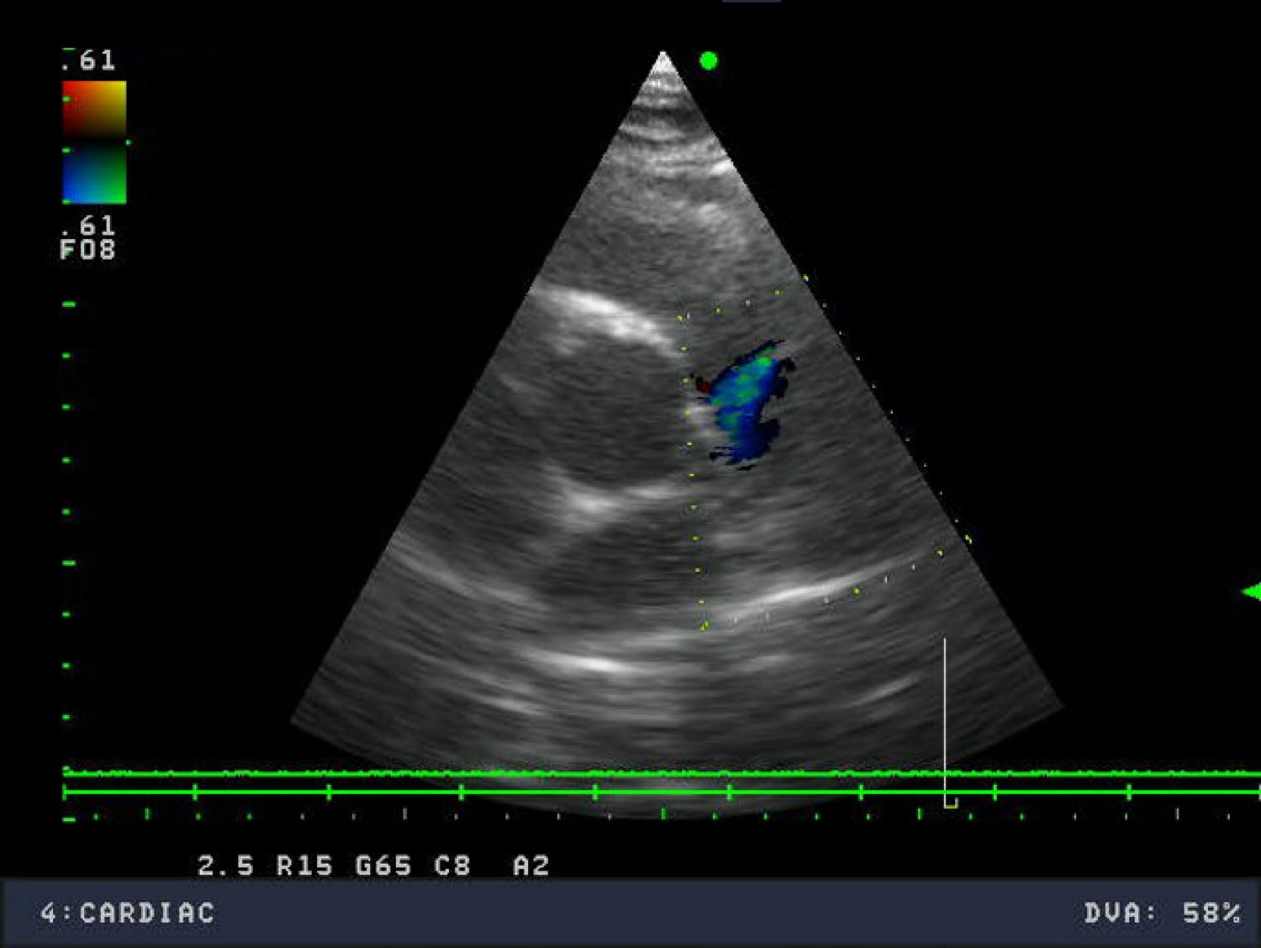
Echocardiography (parasternal short axis view at the level of the great vessels) showing abnormal flow into the pulmonary artery
